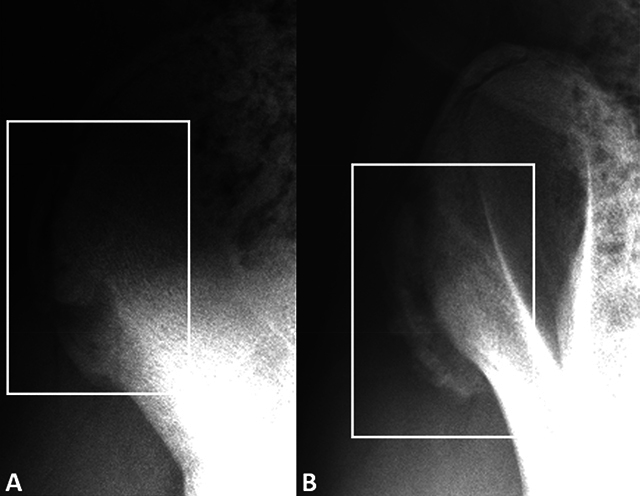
Radiograph of the right hip shows fragmentation and displacement of the anterior third of the ossification center of the iliac crest with periosteal reaction.
